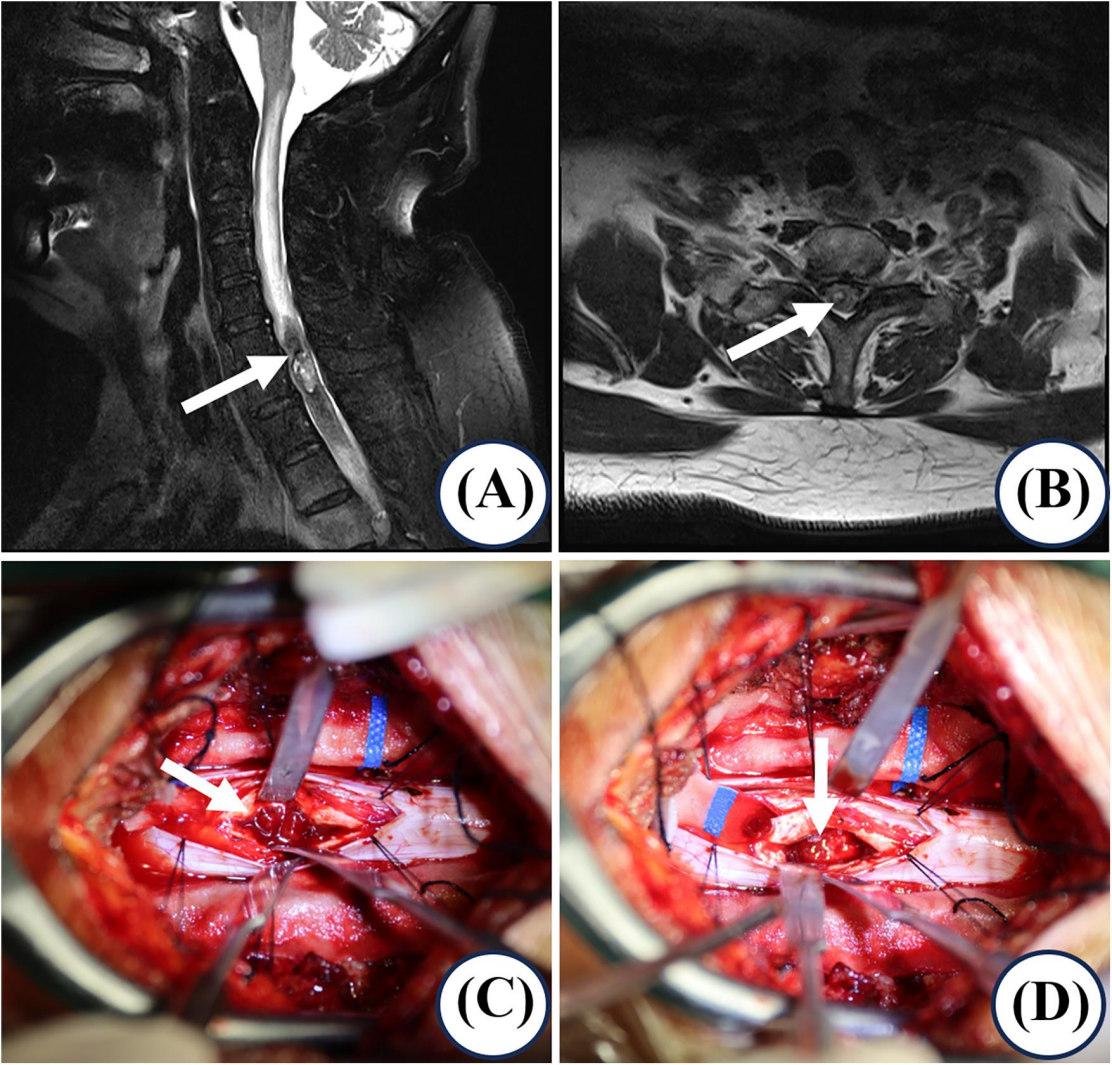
Diagnostic imaging and intraoperative confirmation. (A,B) Preoperative cervical MRI reveals a well-demarcated intramedullary lesion at the C7 level (2.0 × 1.5 × 0.8 cm, measured on radiologic sequences with mild spinal cord edema contributing to minor dimensional differences compared to intraoperative findings), demonstrating heterogeneous isointense to mildly hyperintense signals on T2-weighted imaging with homogeneous moderate enhancement. Characteristic imaging features include a peripheral “flame sign” (arrow in A) and a “central dot sign” on the axial section (arrow in B). (C,D) Intraoperative photographs identify an intradural tumor posterior to the C7 vertebral body, measuring approximately 1.6 cm × 0.8 cm × 0.8 cm (initial gross measurement prior to complete tumor exposure), with a relatively distinct boundary from the spinal cord, soft in texture, and grayish-white in appearance. The tumor was resected in a piecemeal fashion, achieving maximal tumor removal and decompression of the spinal cord.
